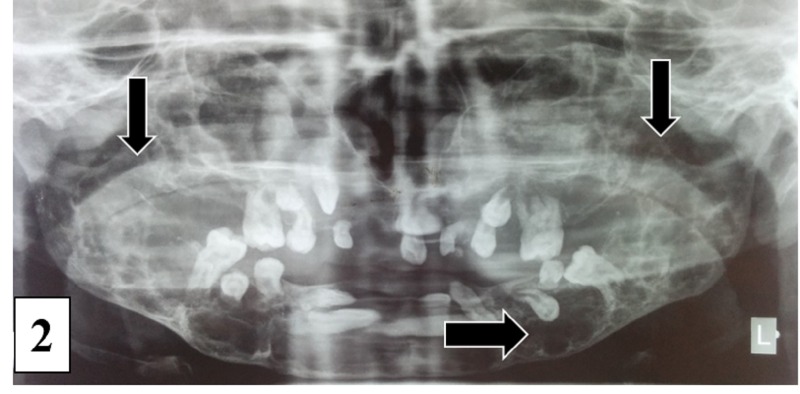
Panoramic Radiograph Multiple radiolucencies involving the maxilla and mandible bilaterally, unerupted and displaced permanent teeth along with involvement of bilateral coronoid process.
